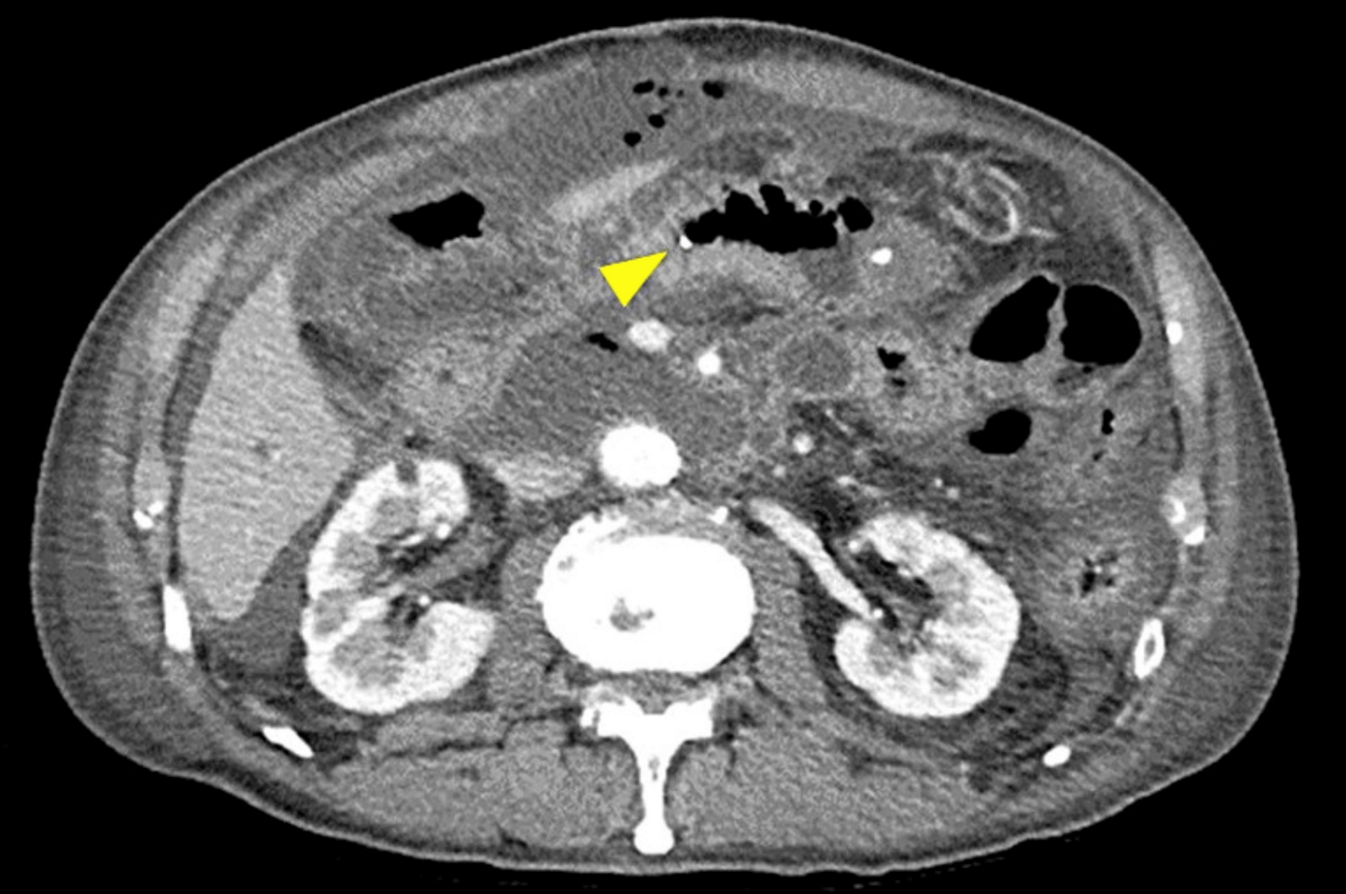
Enhanced abdominal computed tomography of Case 2 performed 11 days after the reoperation. Computed tomography showed the distal end of the pancreatic stent located within the jejunal loop (arrowhead).
